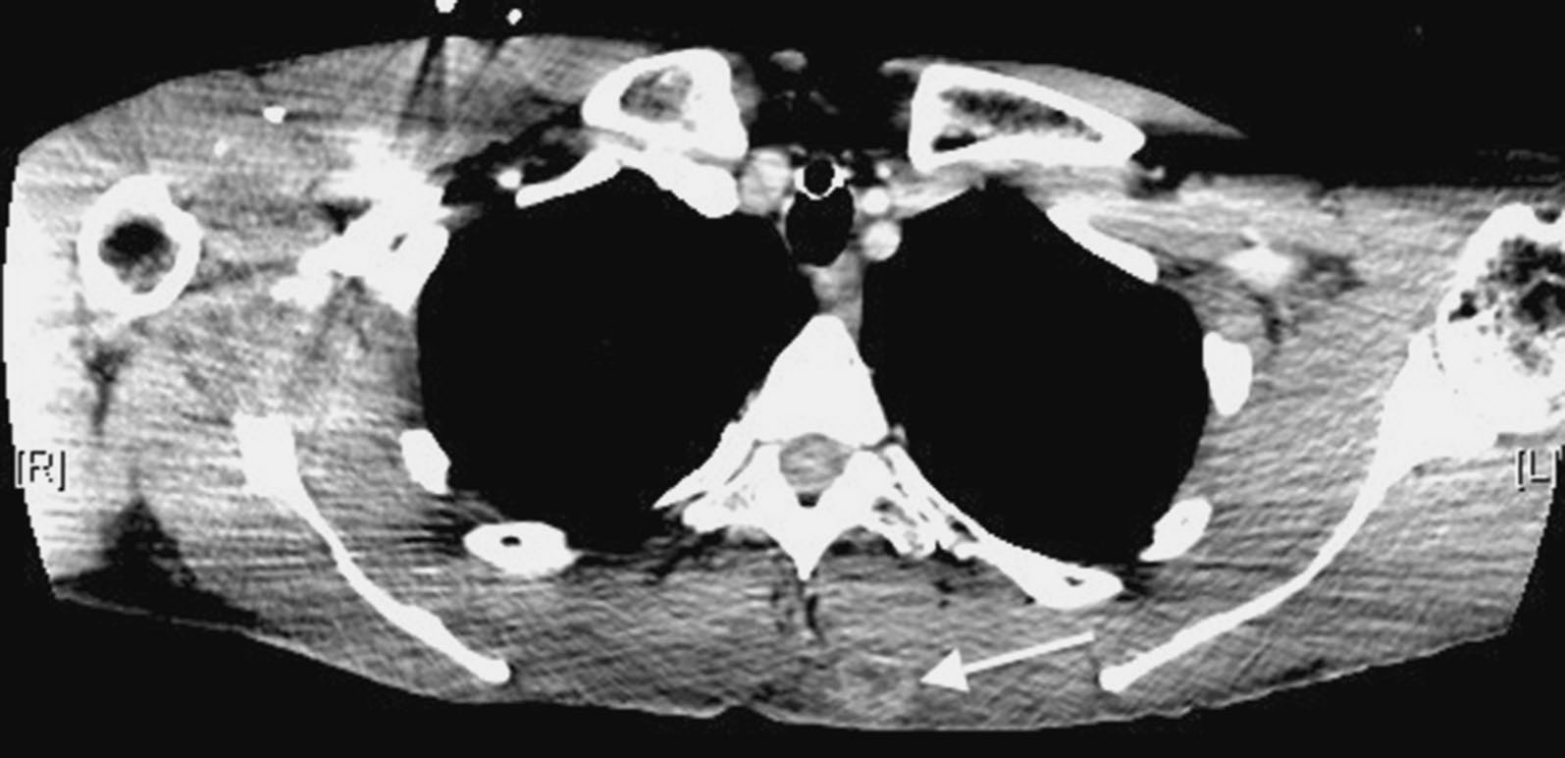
Axial thorax CT post contrast image showing an ill-defined soft tissue mass (*arrow*) superficially located in the left trapezius muscle with peripheral enhancement and low signal in the centre
